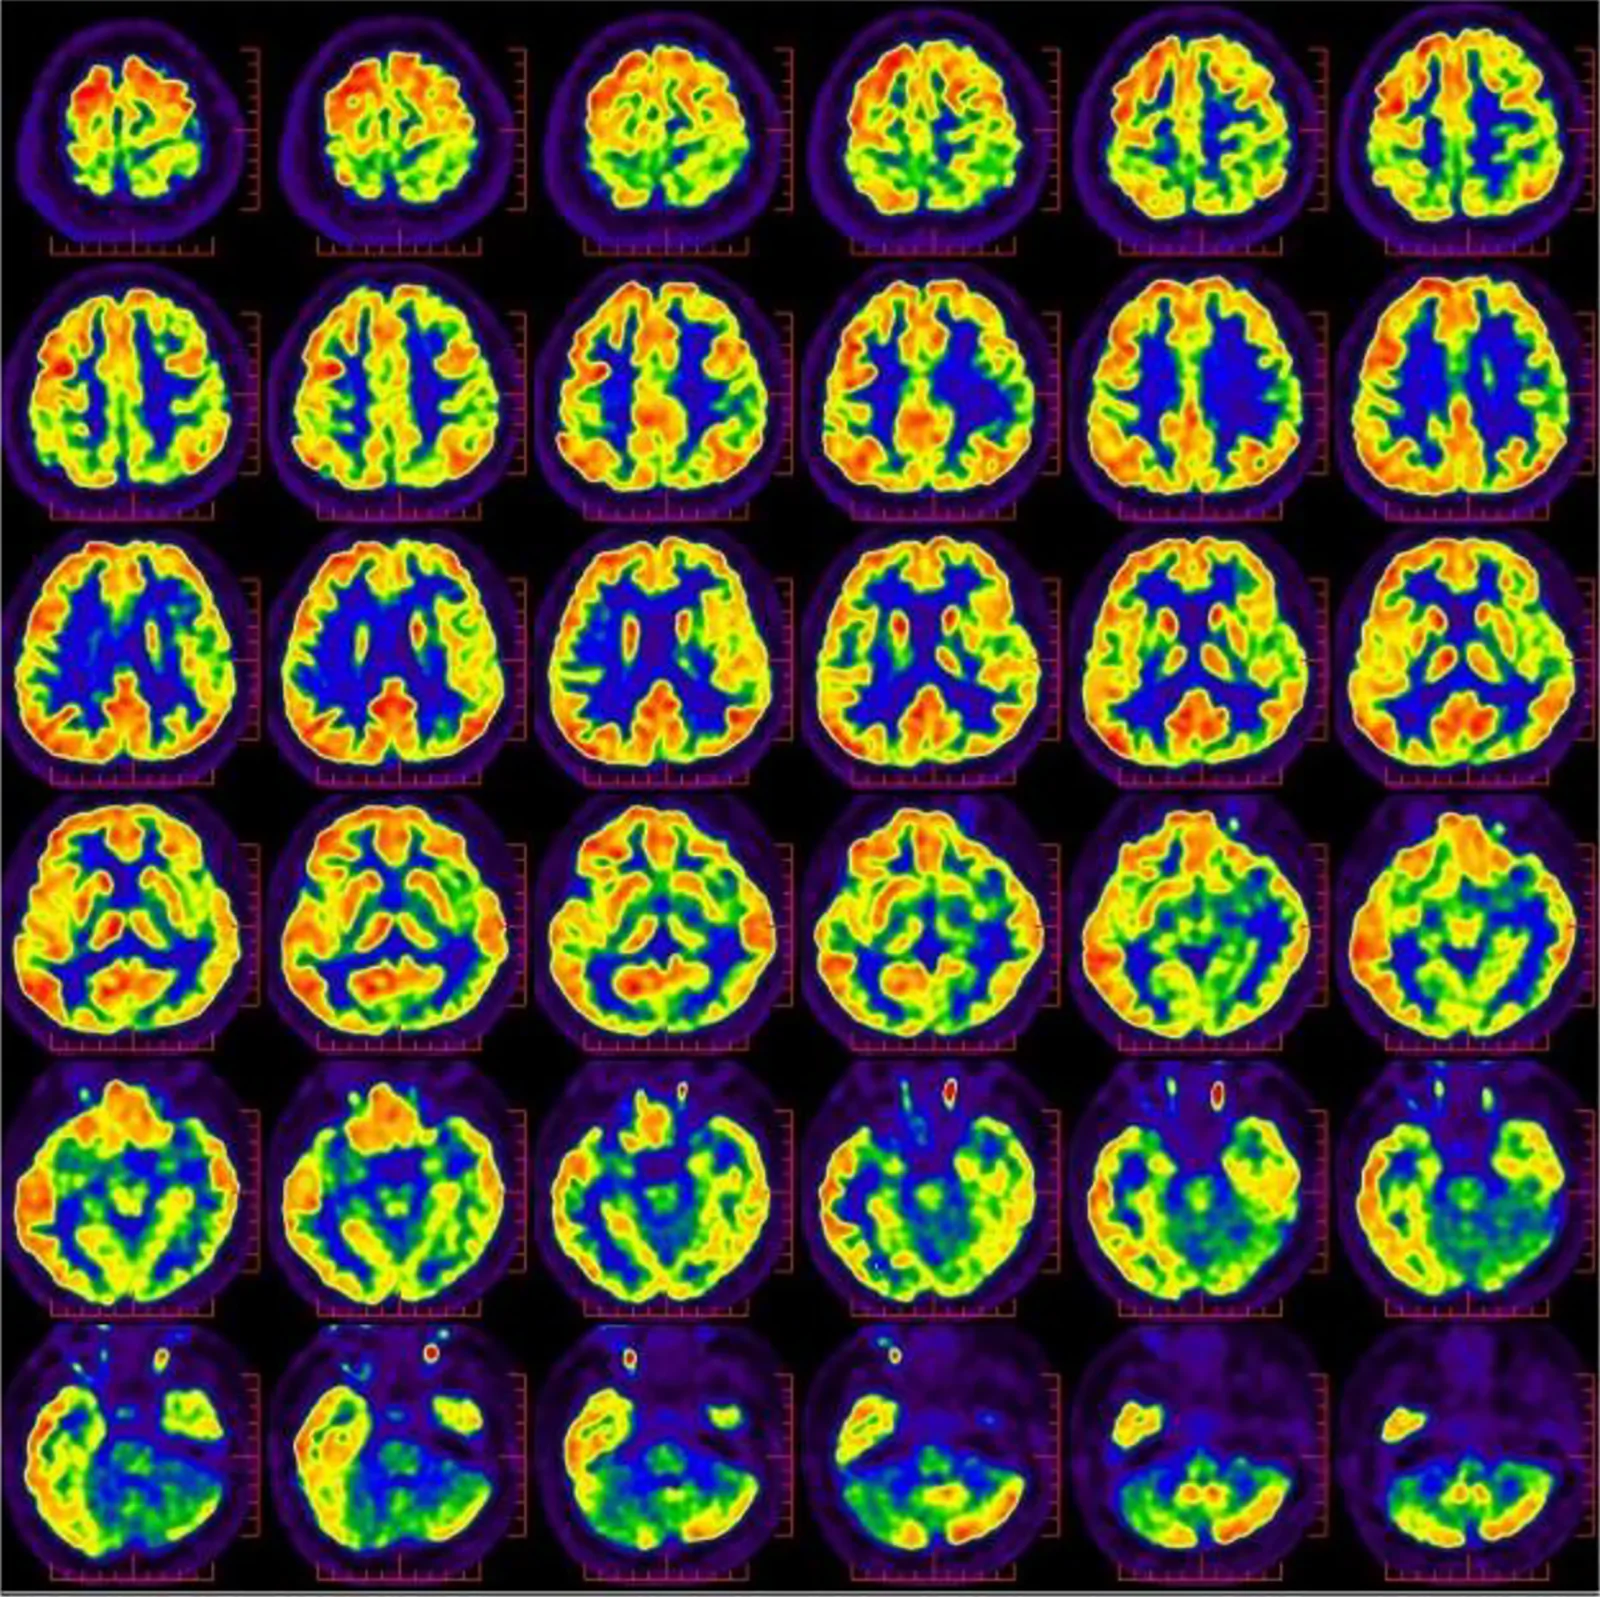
PET/CT showed increased radiotracer uptake in the deep right temporal lobe and right hippocampal region, and decreased uptake in the left fronto-temporo-parieto-occipital lobes and right cerebellar hemisphere. PET/CT = positron emission tomography/computed tomography.
